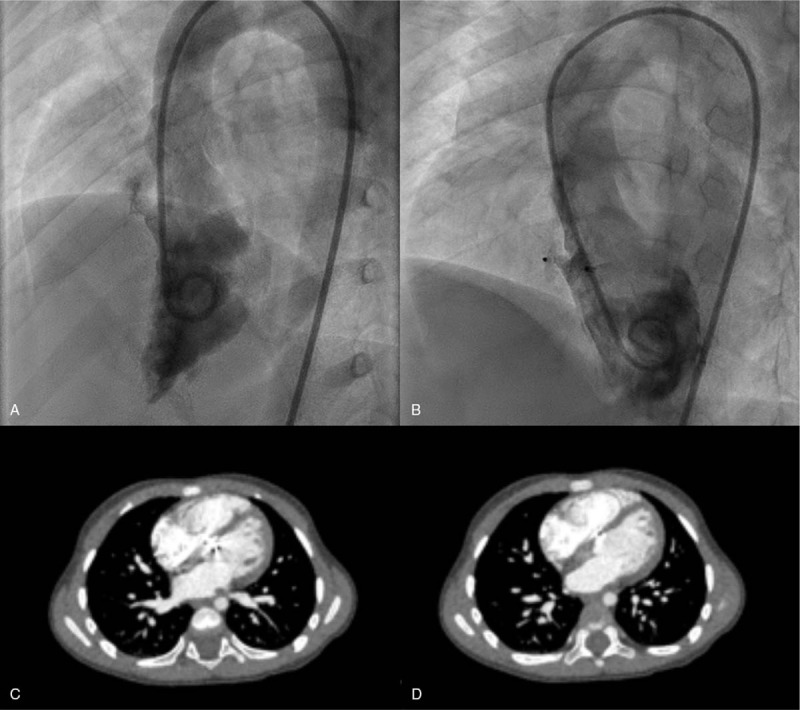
Angiography for during the procedure of LV-RA shunt closure. (A) For the angiography in left ventricle and the right atrium appears at first. (B) For the angiography after closure shows the completed closure of the defect. The CT scanning for the device position. (C and D) Show the different level of heart section. CT = computed tomography, LV-RA = left ventricle-right atrium.
